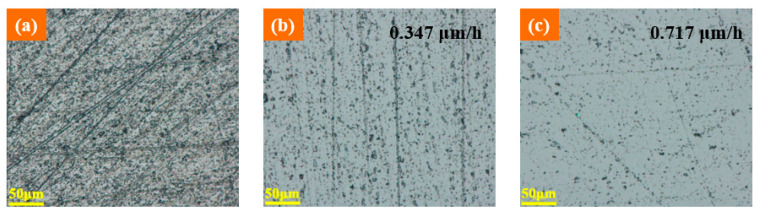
Influence of ultrasonic vibration on CMP: (a) optical image after common lapping; (b) optical image after common CMP after common lapping; (c) optical image after ultrasonic CMP after common lapping.
